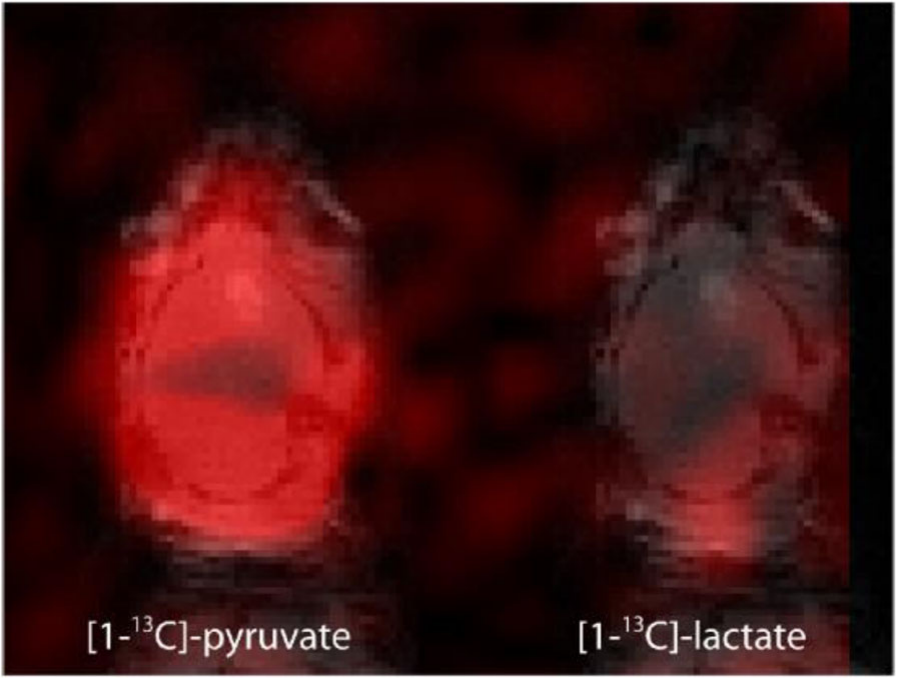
Example of a hyperpolarized pyruvate and lactate image mapped to the T2-weighted reference image (no BBB disruption)
